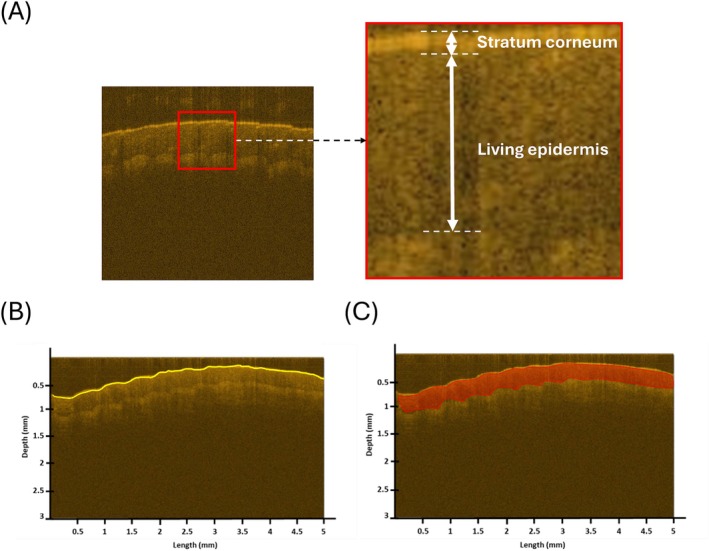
Depth structure of the SC and ED layers. (A) Skin layers, (B) SC image segmentation in yellow, (C) ED image segmentation in red. CT, optical coherence tomography; ED, living epidermis; SC, stratum corneum.
